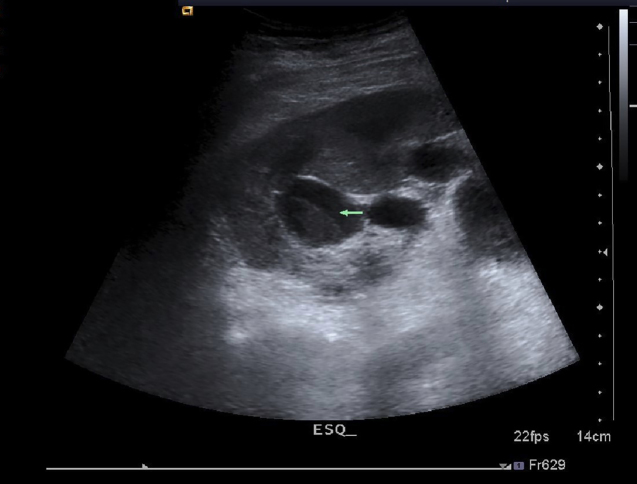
Ultrasonogram of the left kidney showing a hyperechoic mass in the renal calyx with no posterior shadow cone.
